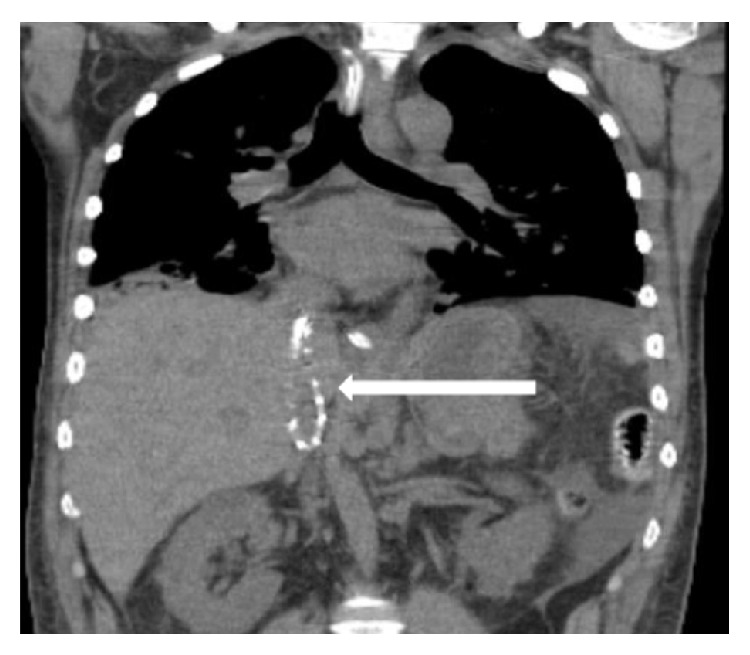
Evidence of retained CVC on predonation CT scan.* White arrow pointing at retained CVC in IVC (coronal view).
